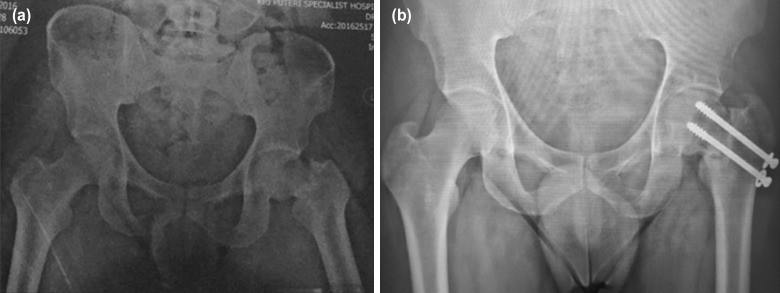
(a) Pelvic radiograph demonstrated left hip basicervical, Pauwels type-3 neck of femur fracture. (b) Pelvic radiograph six months after two cannulated screws fixation, noted non-union over fracture site evidenced by no bridging callus formation and sclerotic fracture edge.
